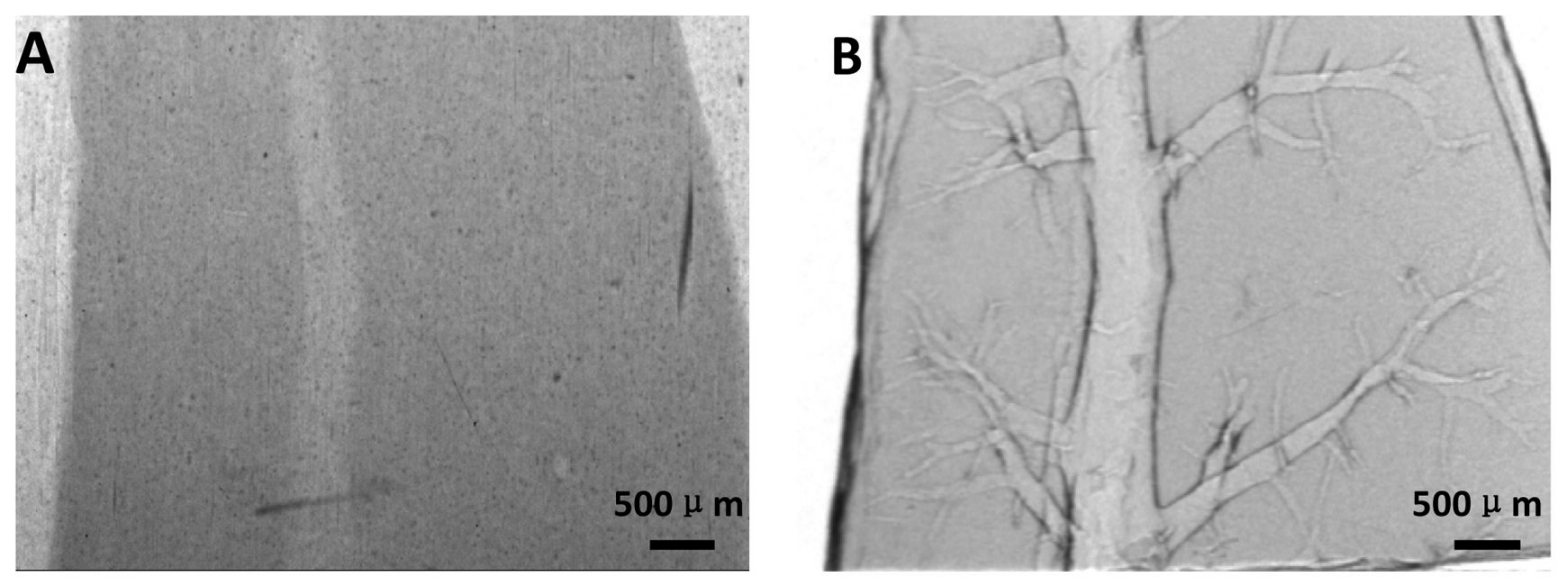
Planar x-ray images of moderate hepatic fibrosis. (A) Conventional radiograph. (B) DEI projection image.
